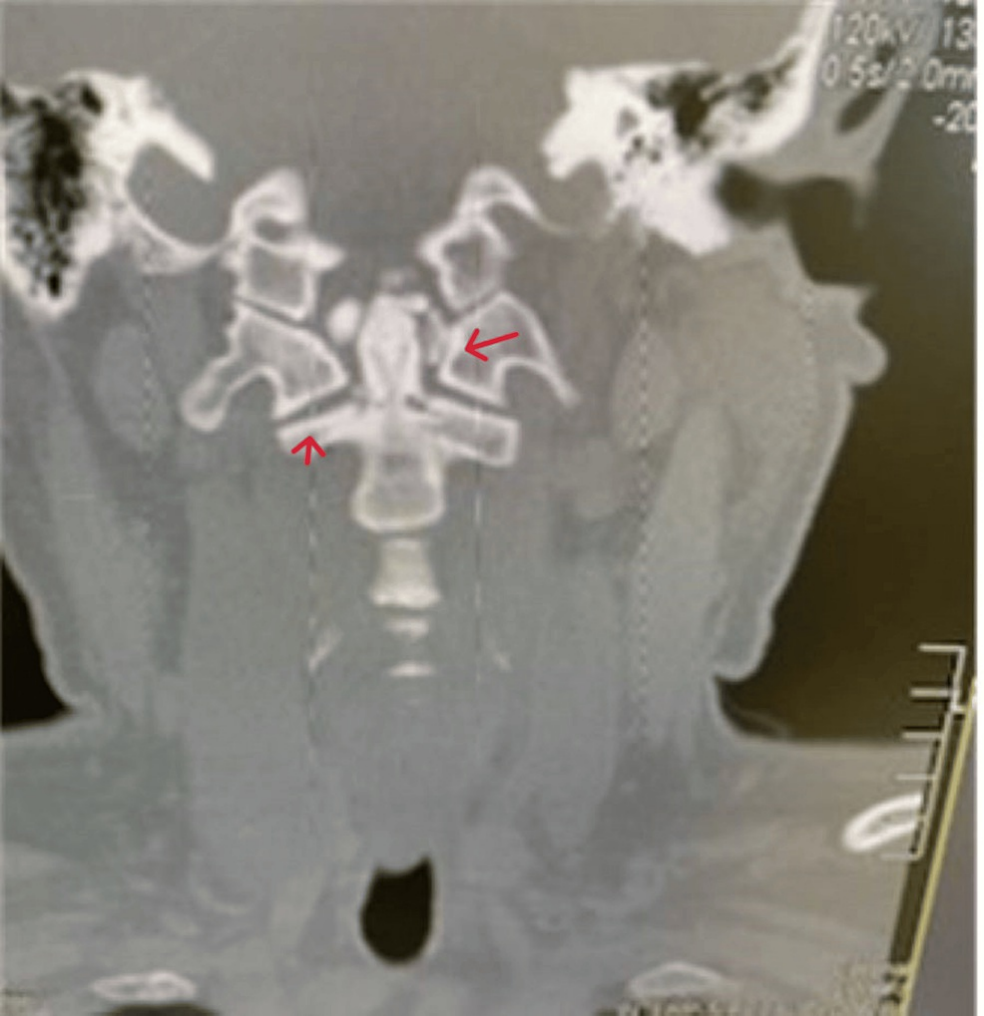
CT (coronal view) of the cervical spine showing spondylolisthesis.
